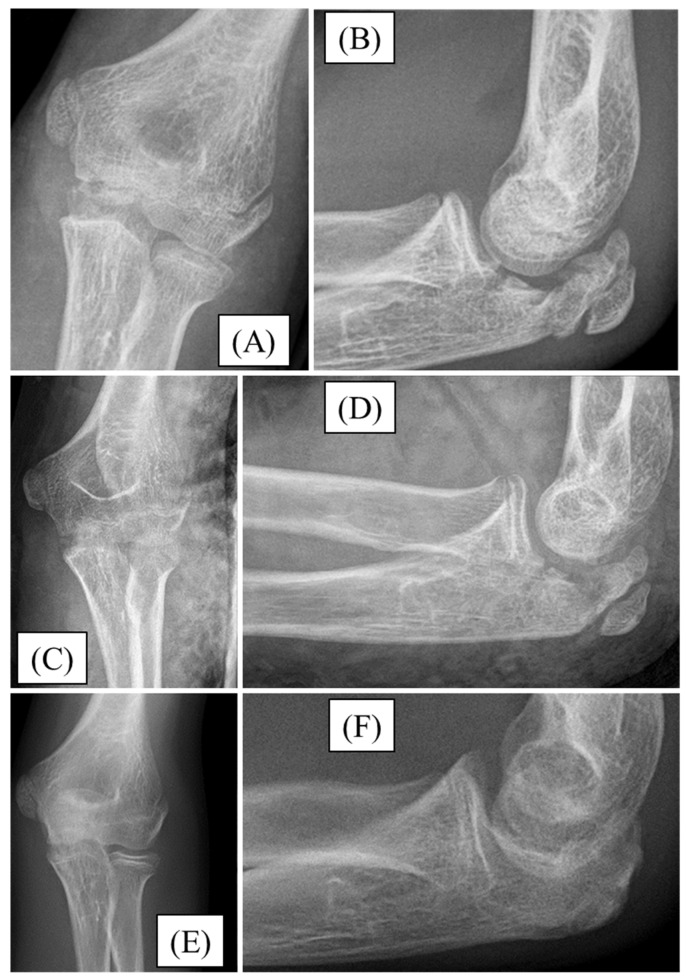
Preoperative X-rays from AP (A) and lateral (B) aspects of olecranon fracture. Postoperative X-rays show good reduction and perfect articular congruency; AP (C) and lateral (D). One year later, the fracture was entirely healed with no sign of articular incongruency (E,F).
